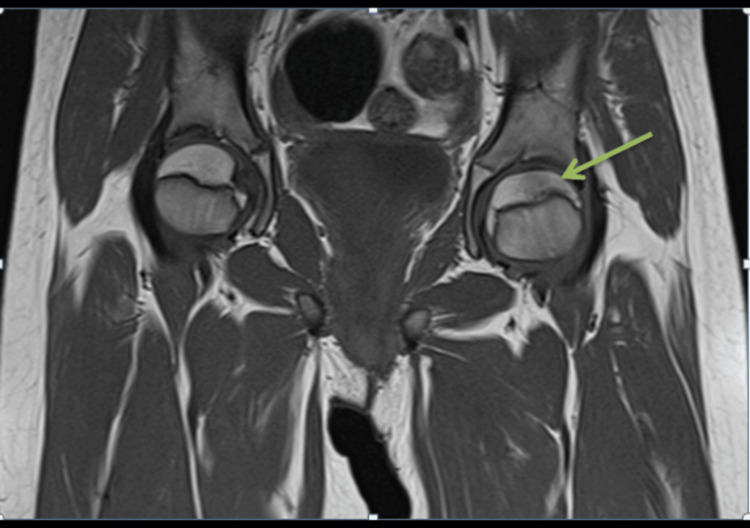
Coronal hip MRI showing a band-like rectangular focal abnormal signal on the left femoral head, which is low on the T1-weighted image (green arrow).
